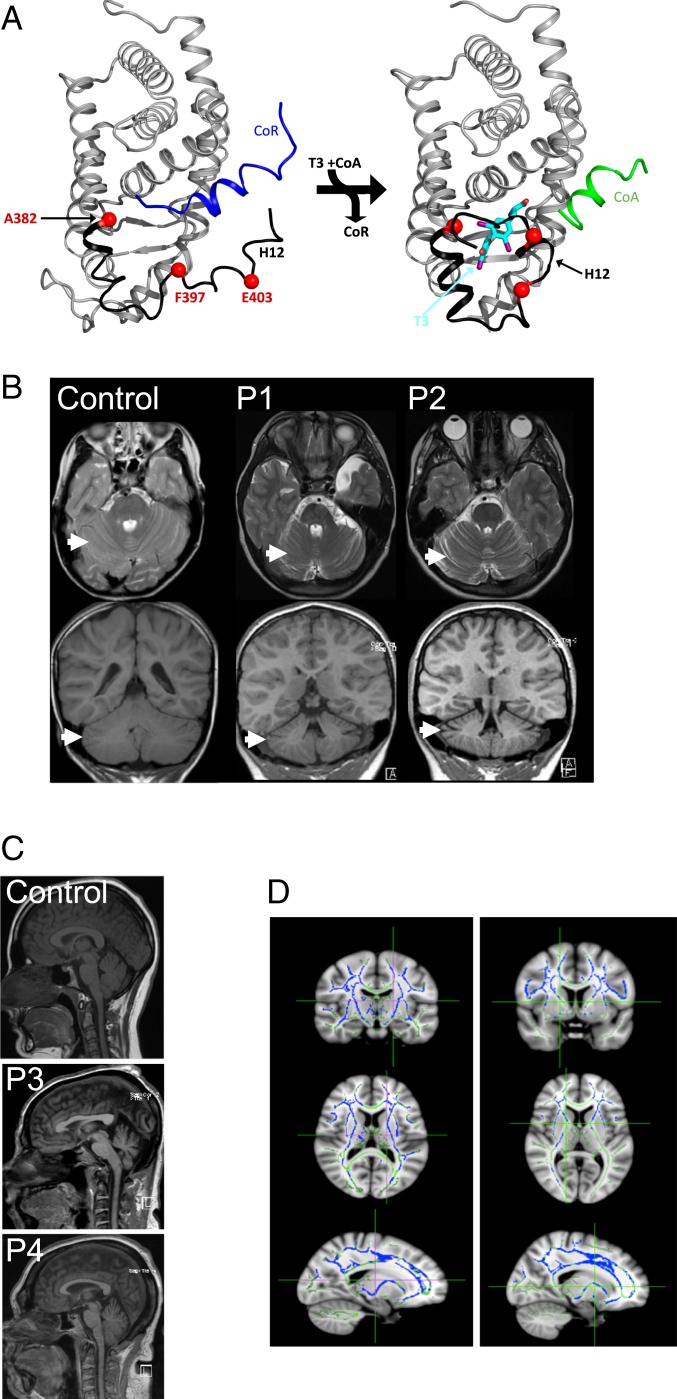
THRA* mutations are associated with structural abnormalities in the brain. (*A*) Structural modeling of the ligand-binding domain of TRα1 showing the position of mutations (red). The mutations in patients P4 (A382PfsX7), P2 and P3 (F397fs406X), and P1 (E403X) disrupt or truncate the carboxyterminal alpha helix (H12; black) of the receptor, exposing a hydrophobic cleft that facilitates corepressor (CoR; blue) binding by unliganded receptor (*Left*) and removing or changing amino acids required for T3 (cyan) binding and coactivator (green) recruitment (*Right*). (*B*) MRI scans of patients P1 and P2 and a control subject (female, age 10 y 8 mo) with T2-weighted axial images (*Top*) and T1-weighted coronal images (*Bottom*), showing increased CSF space (arrows) around the cerebellum and between folia, denoting reduced cerebellar size. (*C*) Sagittal images from MRI brain scans of adult cases P3 and P4 and a control subject (female, age 52 y) showing microencephaly. (*D*) Tract-based spatial statistics analysis of DTI data in patients P1 and P2. Tracts highlighted in blue signify greater mean diffusivity (MD) of water than in controls (*n* = 20 age- and sex-matched subjects), and tracts highlighted in green denote not significantly different MD compared with controls.
